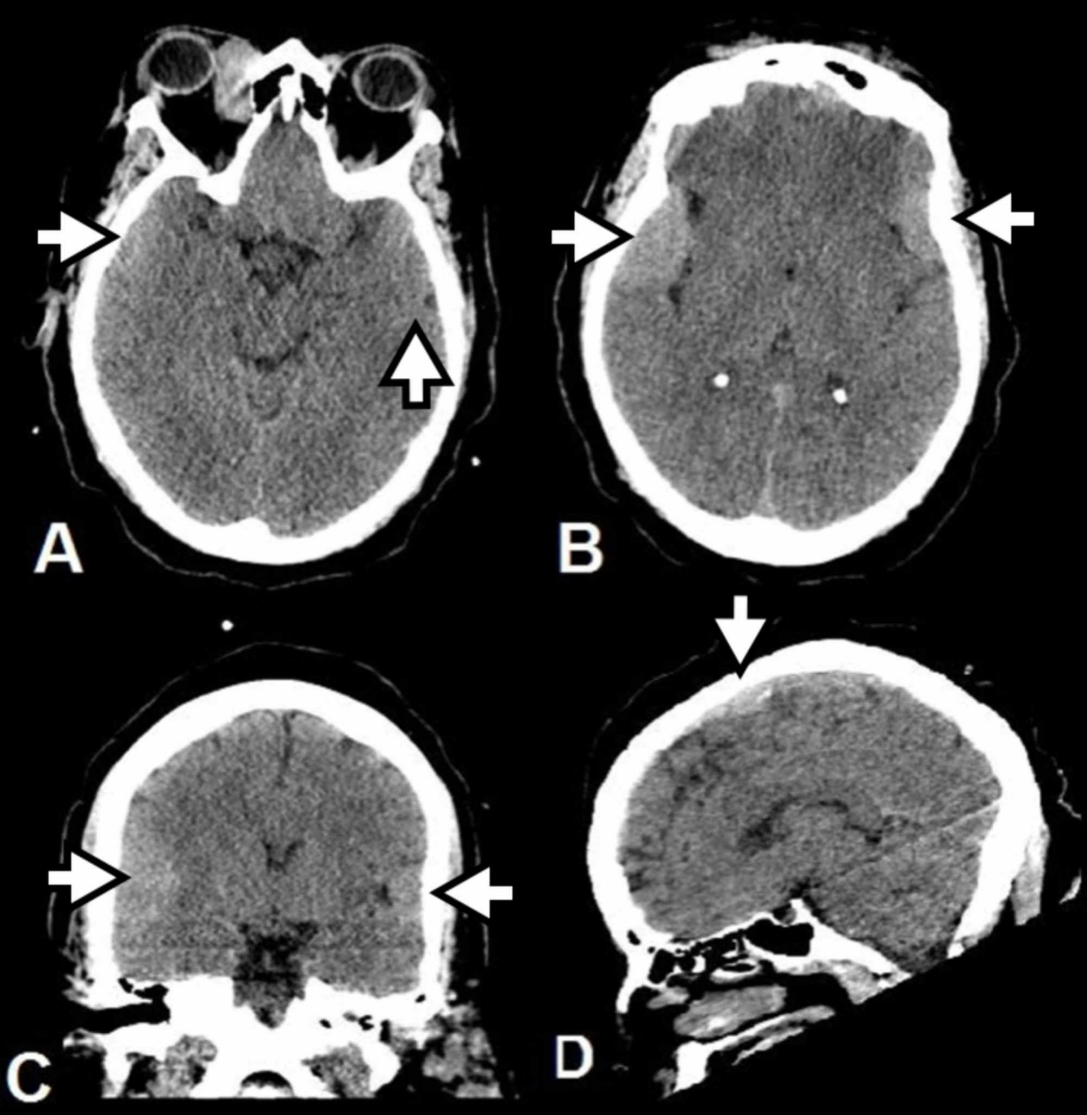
Screening Computed Tomography Head Scans Axial (A&B), coronal (C), and sagittal (D) slices show multiple extra-axial hyperdense masses with effacement of the adjacent sulci and gyri. The largest in the right frontal-temporal region measures 4.0 centimeters anterior-posterior and 3.7 centimeters cranial-caudal.
